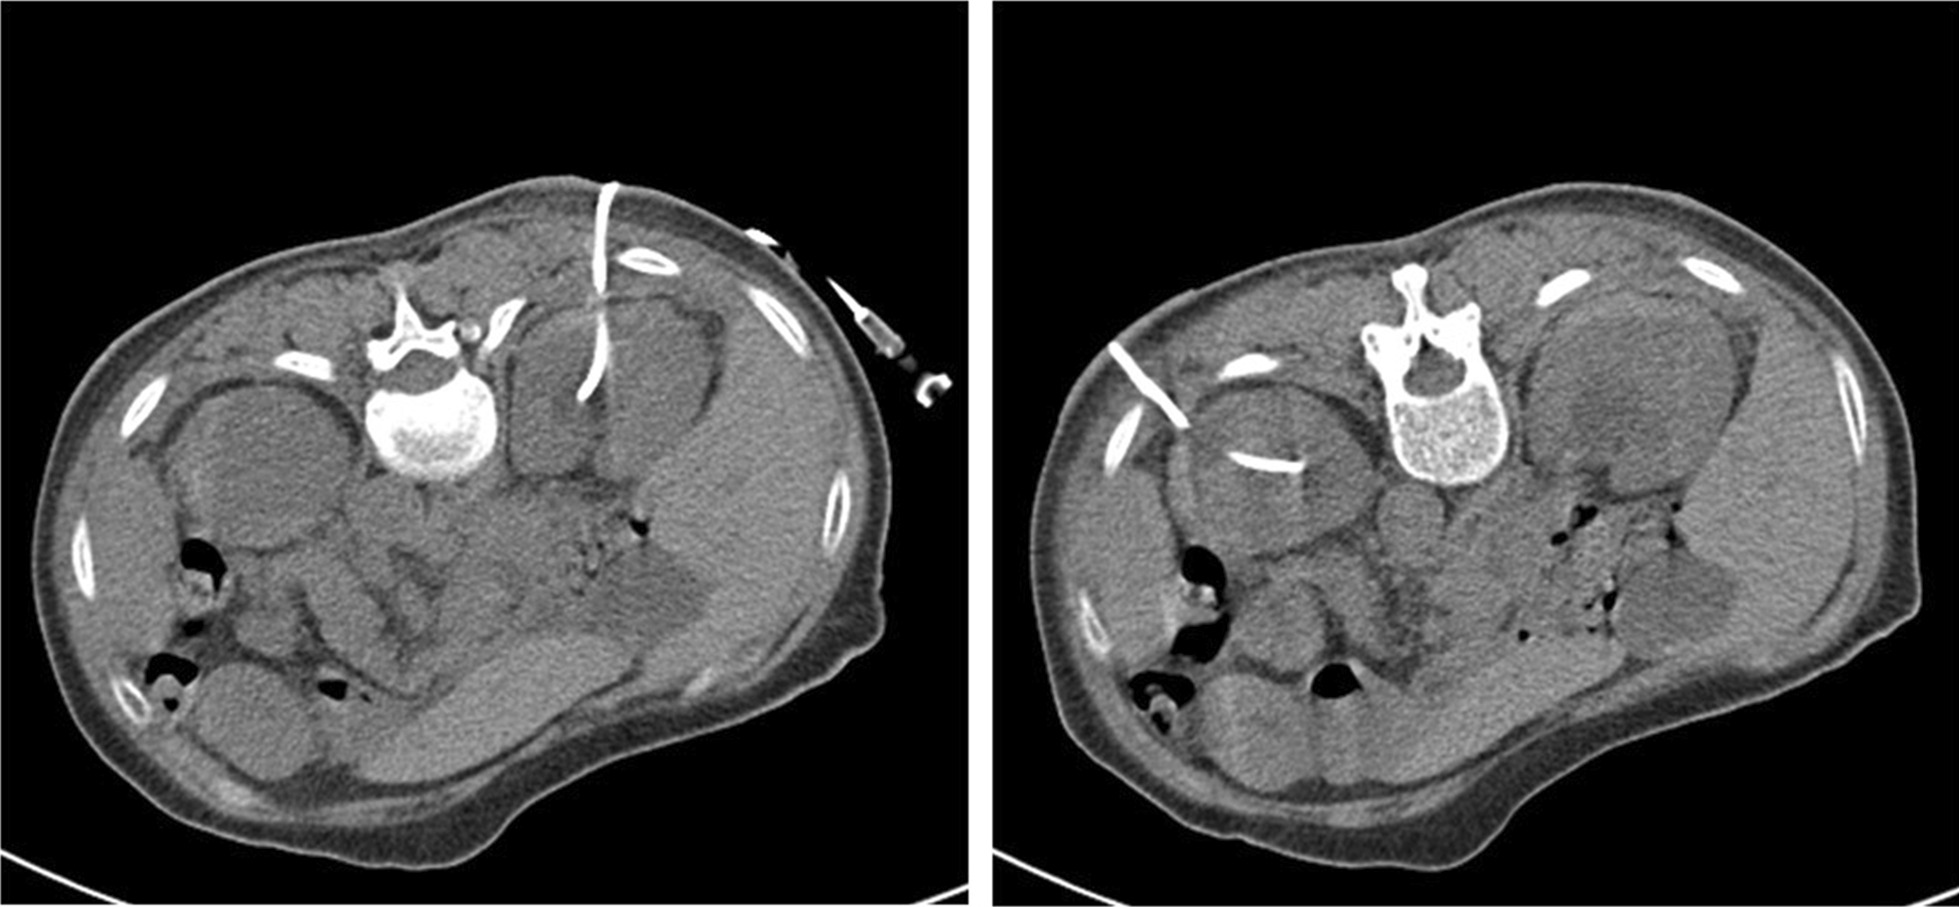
Bilateral computed tomography guided percutaneous nephrostomy with supracostal approach
